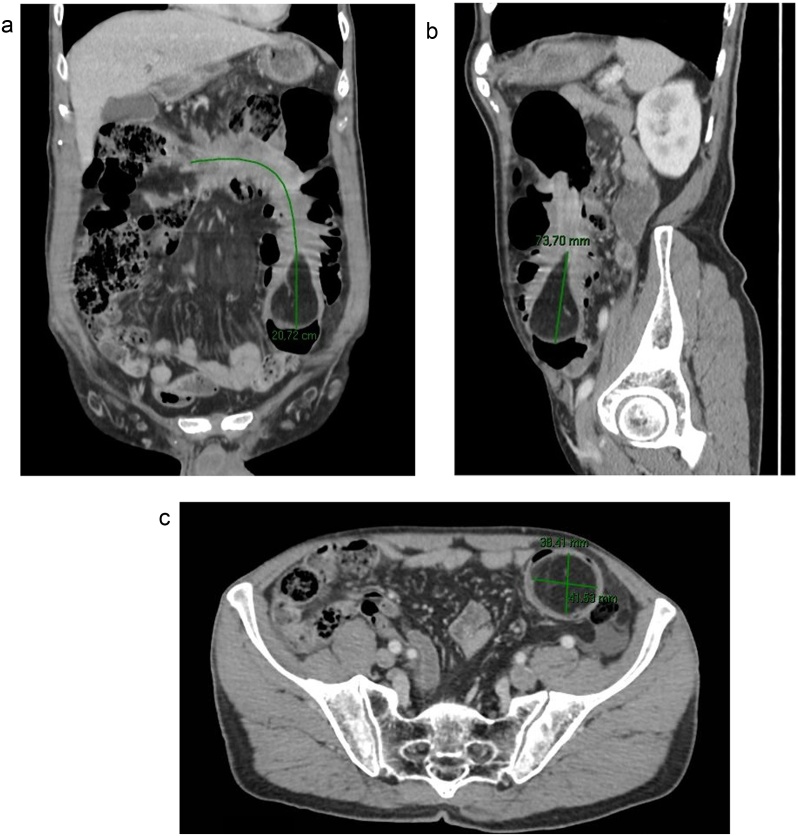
A – CT scan, coronal section in portal phase: Intestinal invagination extending longitudinally by 20.7 cm. The segments involved present normal parietal enhancement by the iodinated contrast media. B – CT scan, sagittal section in portal venous phase: Head of the intussusceptum related to the fat densit, round formation, measuring 7.3 × 3.8 × 4.1 cm. C – CT scan, axial section in portal venous phase: Head of the intussusceptum related to the fat densit, round formation, measuring 7.3 × 3.8 × 4.1 cm.
